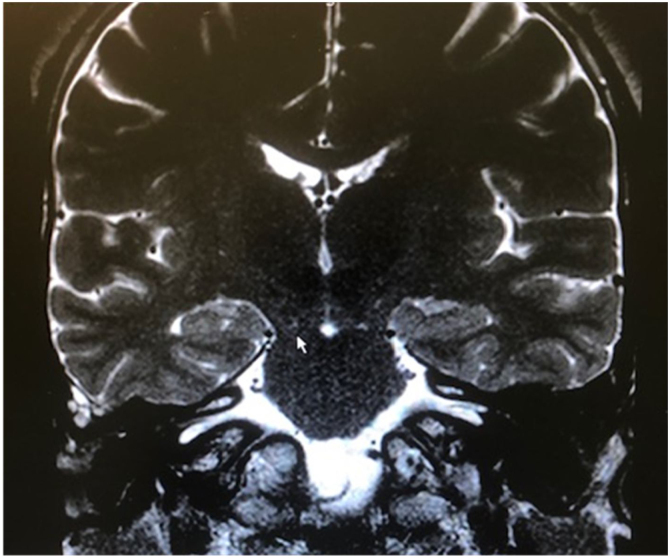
Magnetic resonance imaging (T2-weighted image). Coronal plane. Patient with meningitis. Bilateral ossification of the membranous labyrinth.
